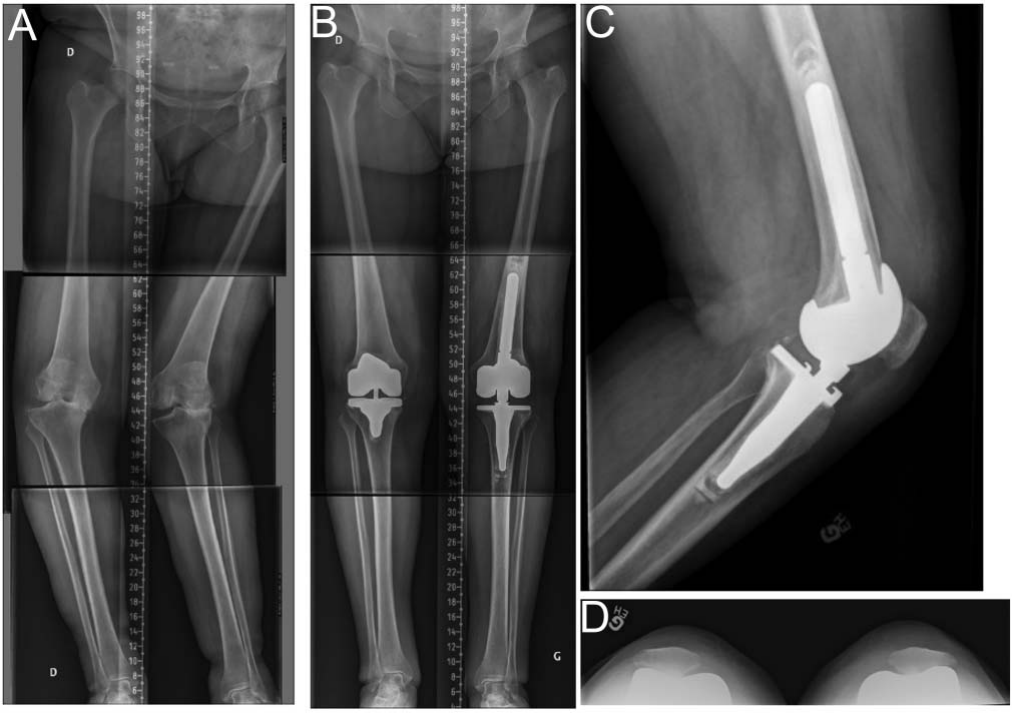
Severe windswept lower limbs. (A) Preoperative long-leg radiographs of a 78-year-old female with a right knee in severe varus (mechanical HKA of 21° degrees) and a left knee in severe valgus (mechanical HKA of 28° valgus). (B, C, D) Using the rKA protocol, the right knee was left with an imbalance of 4 mm after MCL release and required a semi-constrained insert. On the left side, the MCL was incompetent, and a hinge TKA was implanted.
